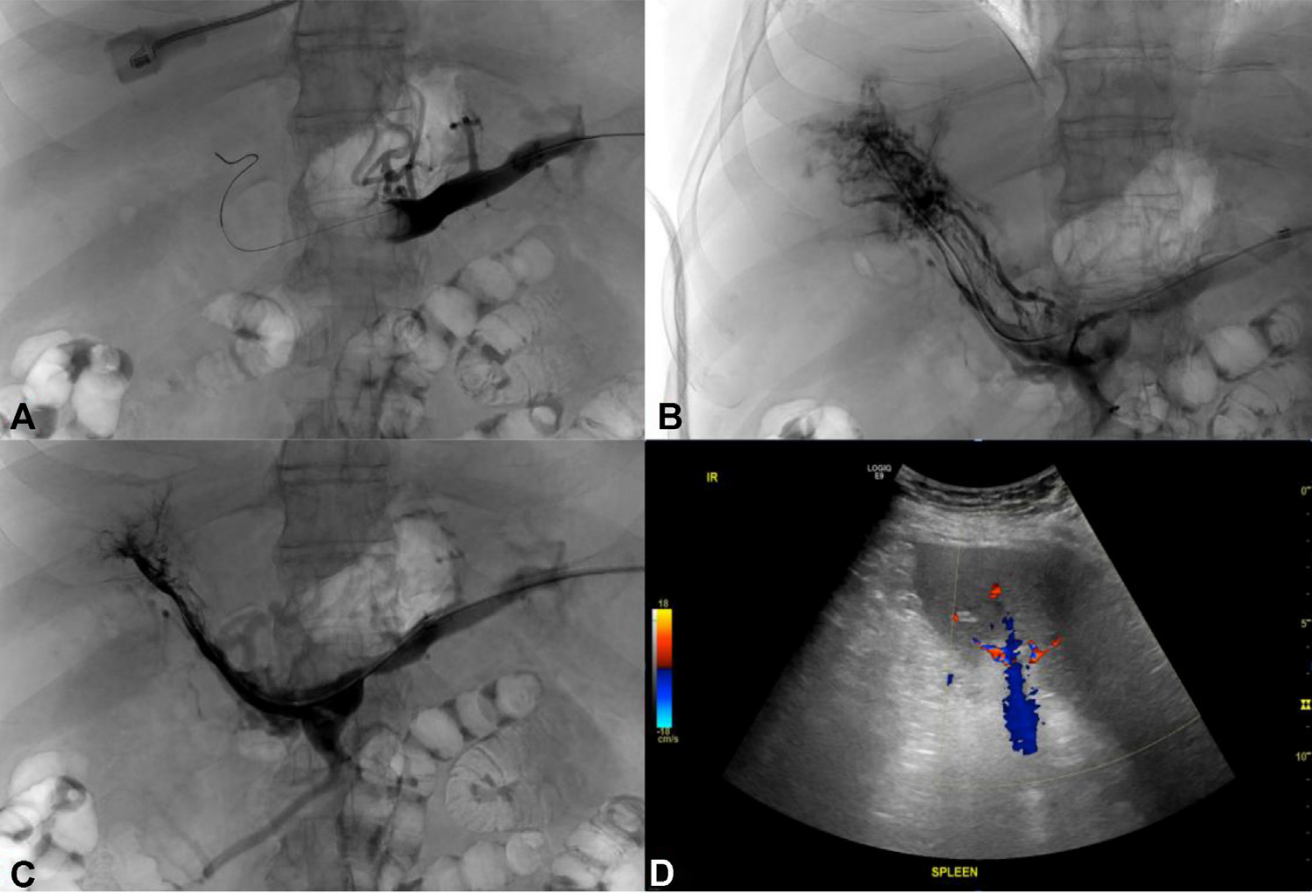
Portal vein thrombus recanalization using TSA. (A) The splenic vein was accessed using a 21-gauge micropuncture needle followed by a 0.018 inch guidewire and eventual transitional dilator once the needle was removed. Next, an 8 French sheath was selected and placed into the splenic vein, and venography of the splenic and portal veins was performed, demonstrating large, nonocclusive portal vein and splenic vein thrombi. (B) After identifying thrombi within the portal and splenic veins, an 8 French Aspirex mechanical thrombectomy device (Bard Peripheral Vascular, Tempe, Arizona, USA) was advanced over a 0.035 inch guidewire to perform mechanical aspiration thrombectomy. Repeat venography demonstrated improved vascular flow. (C) Repeat thrombectomy was done to further decrease clot burden and improve portal and splenic vein flow, allowing for a TIPS stent-graft to be placed and (D) Scout ultrasound images of the spleen showing patent splenic vein branches within the splenic hilum.
